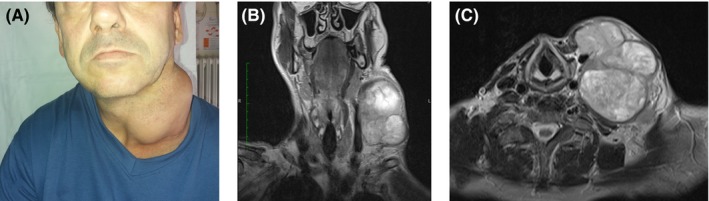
(A) A large solid mass is seen in the left portion of the neck. Coronal (B) and axial (C) contrast‐enhanced magnetic resonance imaging scan showing a T1‐hyperintense solid neck mass
